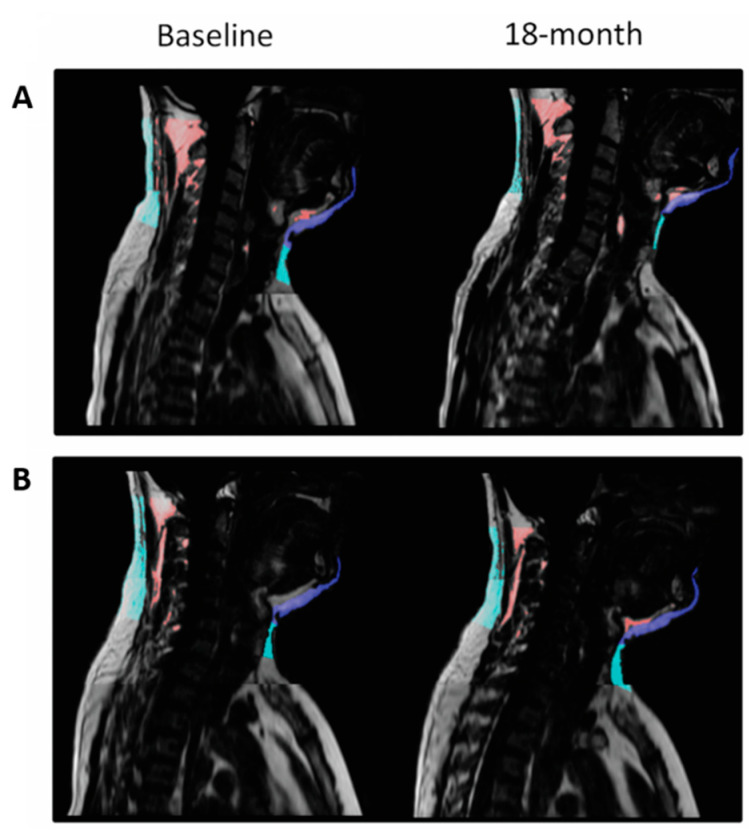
MRI of cervical-SAT—comparison between two matched participants with different weight loss achievements. Panel (A) and (B) demonstrate the sagittal section of subjects A and B, respectively. Cervical-SAT was divided into color-coded compartments: light blue, cervical-SAT; dark blue, chin-SAT; and red, non-classified fat- fat beneath the fascia. Both men had a baseline weight of 83 kg and waist circumference of 105 cm; following the 18-month intervention, participant A lost 18 kg, and participant B gained 5.4 kg. The two men differed distinctly in their 18-month cervical-SAT (−51.97% vs. +2.52%) and chin-SAT (−27.5% vs. +4.82%) reductions.
